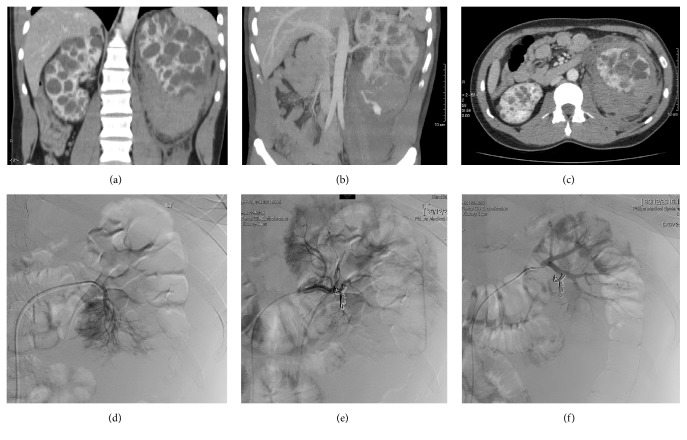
Computerised tomography (CT) of abdomen and left renal arteriogram and embolization of a patient with autosomal dominant polycystic kidney disease demonstrating large left renal retroperitoneal haemorrhage*. Sagittal views (a and b) and (c) axial view of contrast enhanced CT abdomen showing bilateral polycystic kidneys with evidence of large volume haemorrhage which surrounds the left kidney and extends into the retroperitoneal space. Super-selective angiogram of the left renal artery lower polar segmental branch showing an extravasation into the lower polar renal cyst (d) and final, postembolization angiogram confirming satisfactory exclusion of the source of bleed (e and f).
